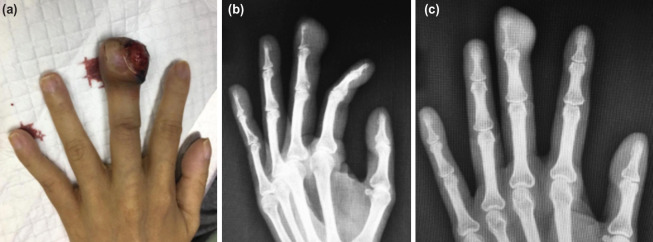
(a) Clinical picture of the left middle finger at three and a half months after onset of symptoms showing fungating mass at the radio-dorsal aspect of the middle finger extending to the distal crease, (b and c) AP and oblique radiograph of the left middle finger showing an eccentric lytic lesion not extending to the distal interphalangeal joint with increase in soft tissue shadow at the distal phalanx.
